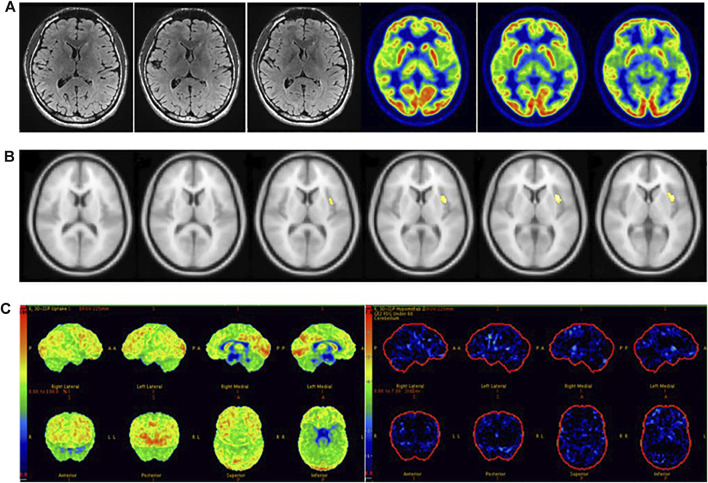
Male, 22 years old, left insula epilepsy. (A) No structural lesions causing seizures were found at MRI 3.0 T epilepsy-protocol. ^18^F-FDG PET showed doubtful abnormal decreased metabolism in the left insula lobe. (B) SPM did not find abnormal metabolic regions whlie *p* < 0.005 and K > 200 but obtain abnormal metabolism in left insula while *p* < 0.005 and K > 50. (C) 3D-SSP did not obtain abnormal metabolic regions. The left insula was surgically resected and lack of seizures for 2 years follow-up.
